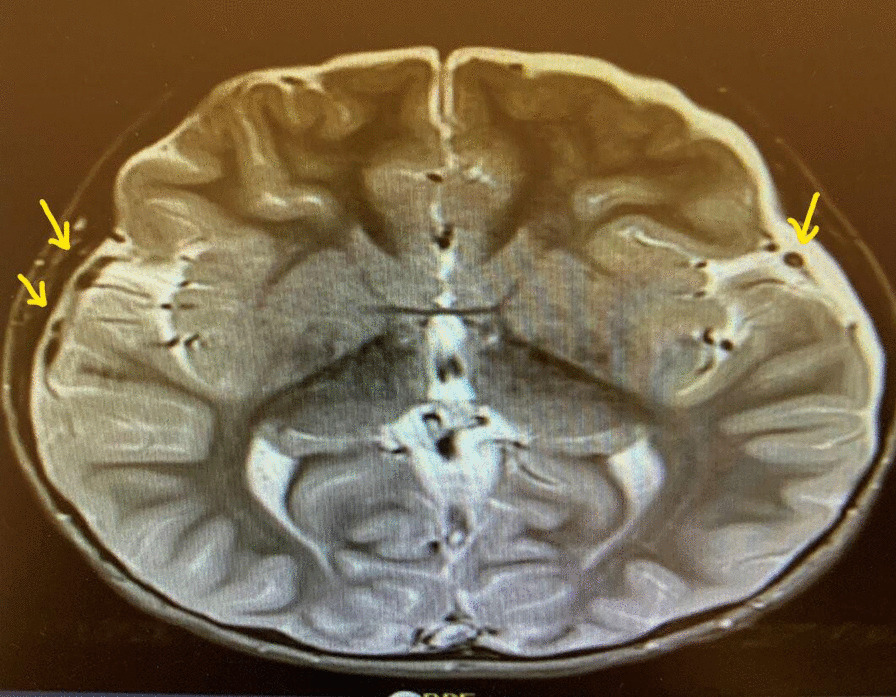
Magnetic resonance imaging (MRI) head - arrows pointing towards pockets of pus
